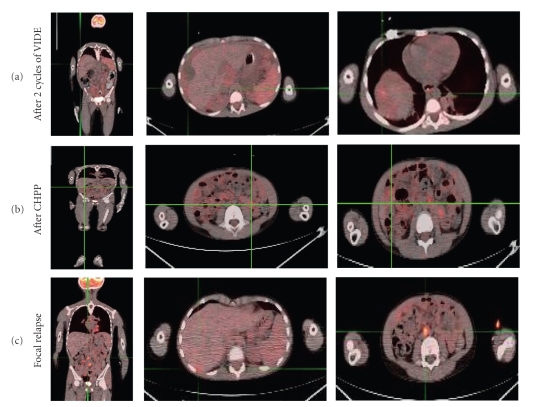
PET-CT fusion imaging*. (a) Residual uptake
